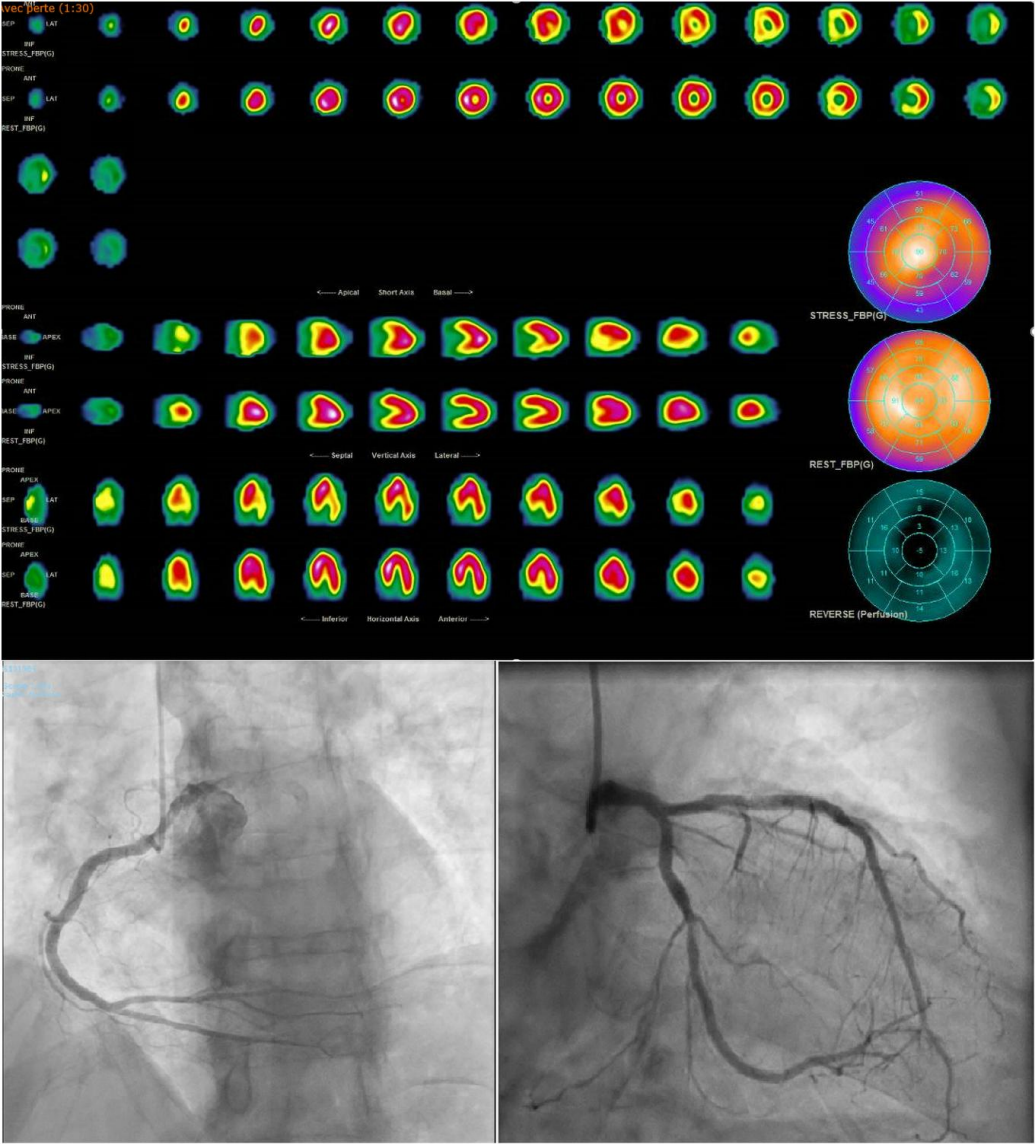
Myocardial perfusion imaging using SPECT in a 79-year-old post-LAD stent patient showing appearance of a reversible inferior and inferolateral ischaemia due to mid-circumflex stenosis, successfully treated by PCI.
